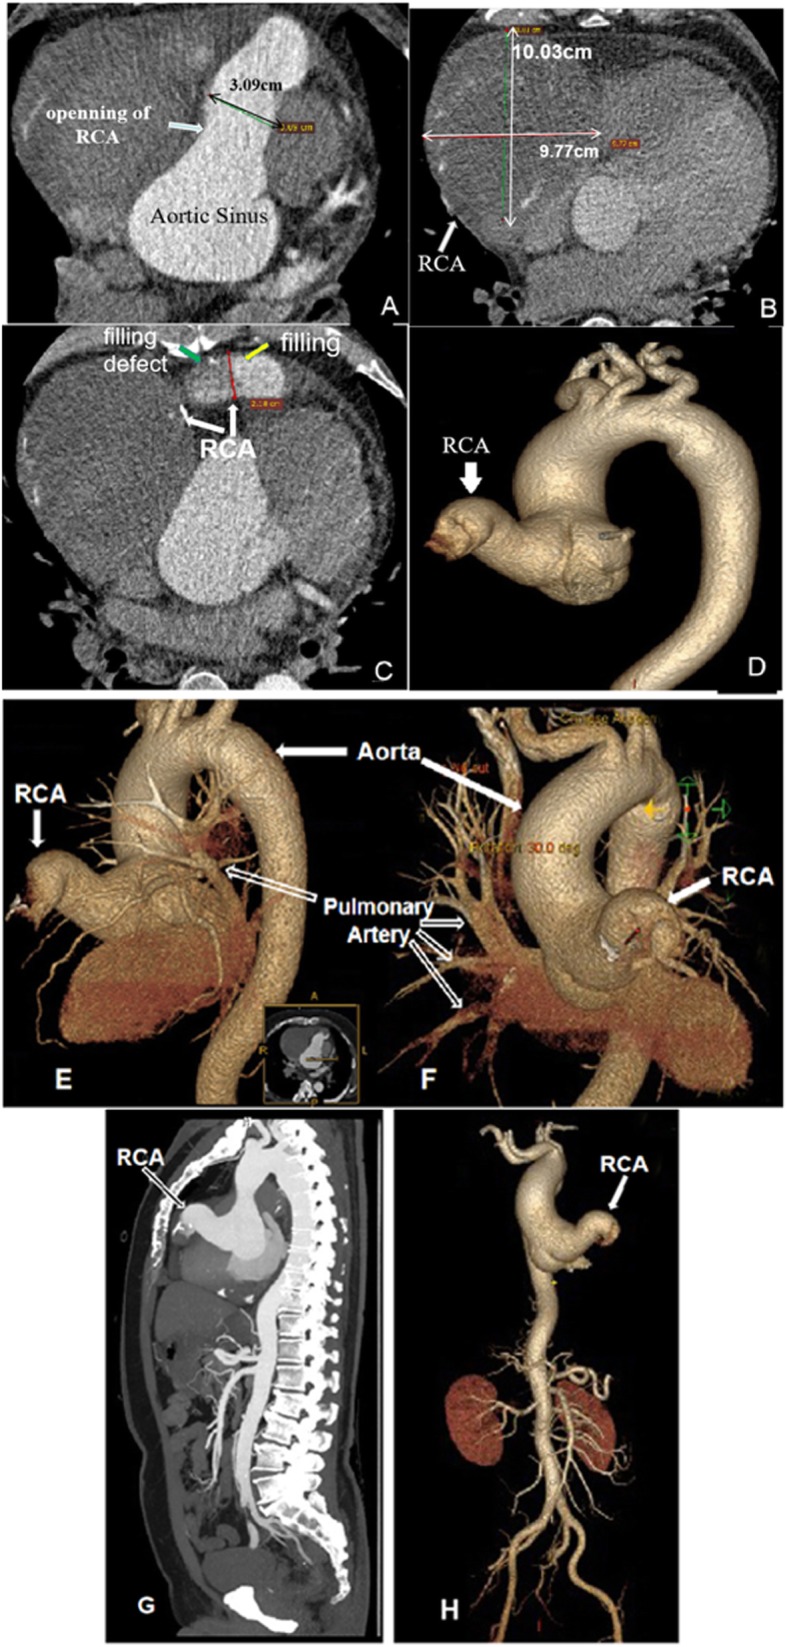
a-d Coronary computed tomography. CT (coronary view) showing origin of the dilated coronary artery from the aortic sinus and the opening of RCA is 3.09 cm (a); the widest part of RCA is more than 10 cm (b); the thrombus blocked the RCA (c) and 3D reconstruction of the heart showing dilated RCA originated from the ascending aorta (d). (e-h) Coronary computed tomography. 3D reconstruction of the heart and great vessels.
